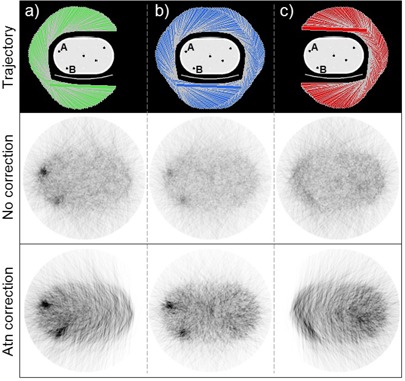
Images from the 4.5 min SPECT scans are displayed beneath their respective detector trajectories. Rows separate images reconstructed with and without attenuation correction. This particular axial slice includes targets A and B.
